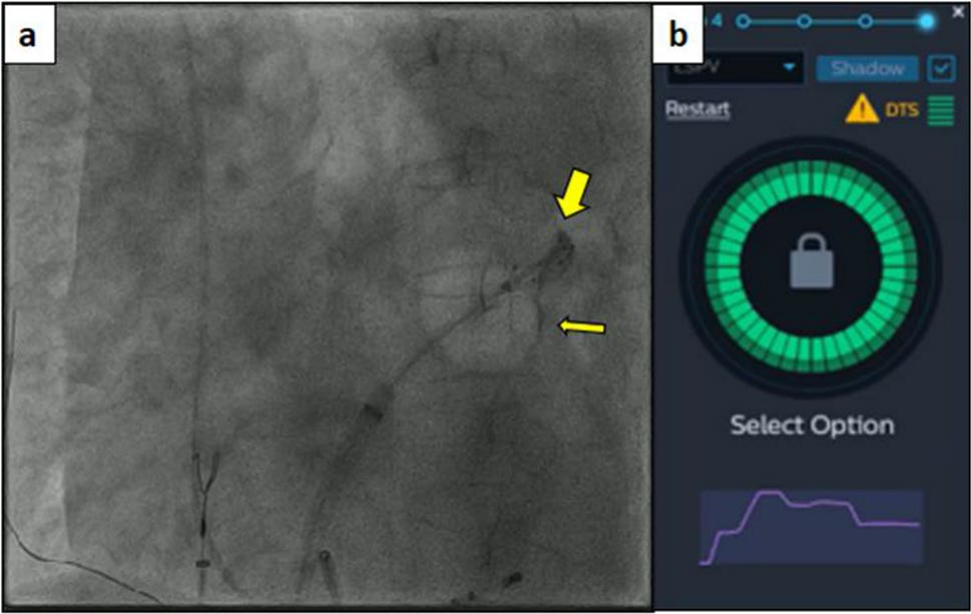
PST under the guidance of KODEX-EPD: (a) retention and leakage of contrast medium in PV under X-ray; (b) changes of dissipation curve of KODEX-EPD injection tool.
